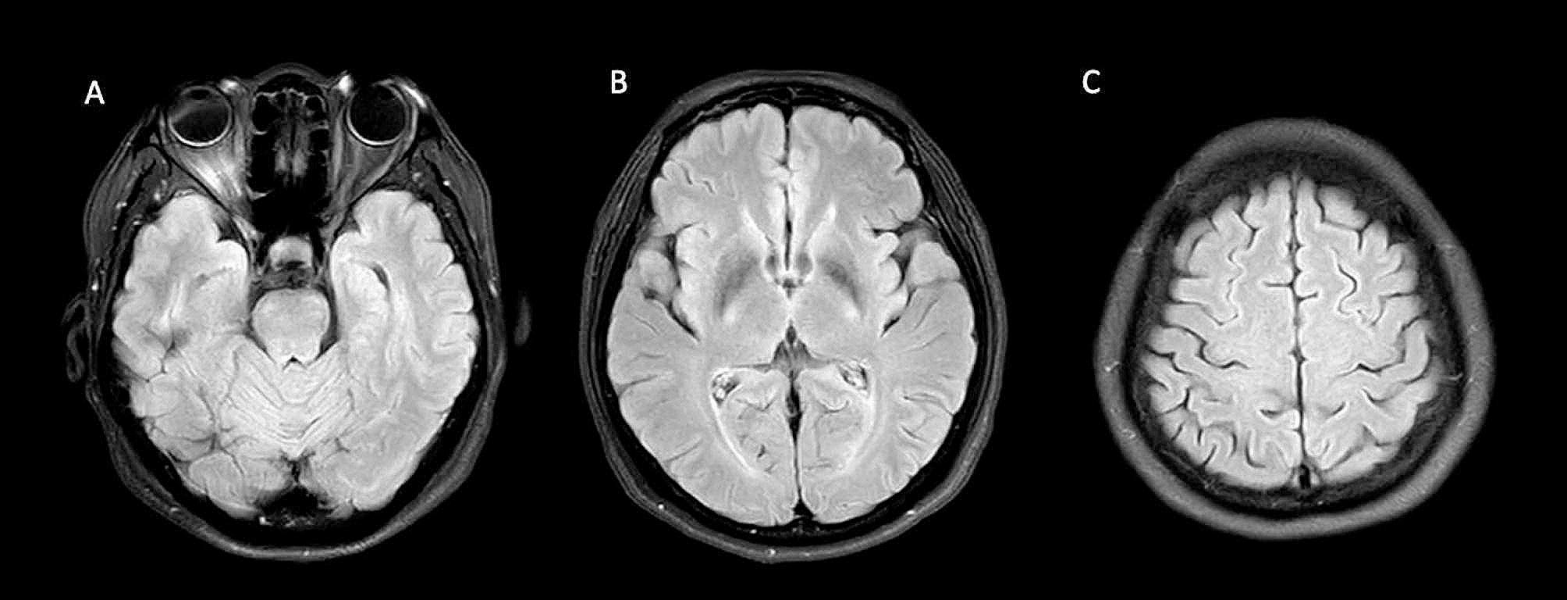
shows magnetic resonance imaging of the brain six months after plasmapheresis, displaying resolving signal intensity on FLAIR in the pons (A), bilateral basal ganglia (B), and the bilateral hand knob area (C)
